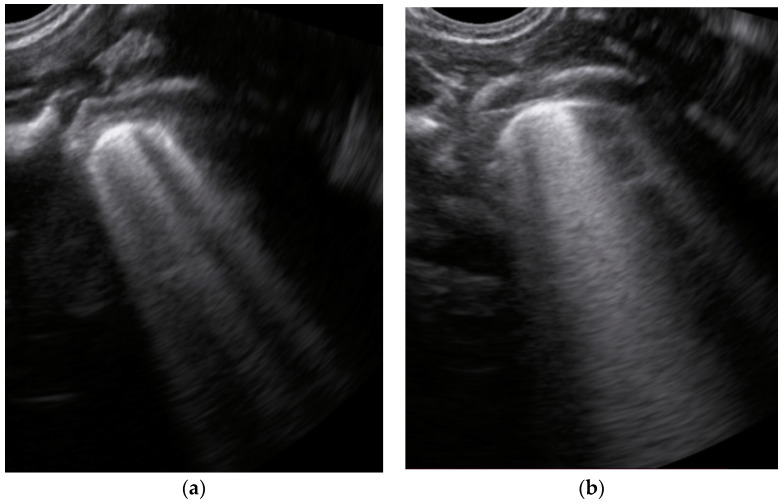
The lung ultrasound showed (a) sparse B-lines with small zones of pleural irregularities corresponding to a LUSS = 1; (b) confluent B-lines with aspect of ‘white-lung ‘corresponding to LUSS = 2.
